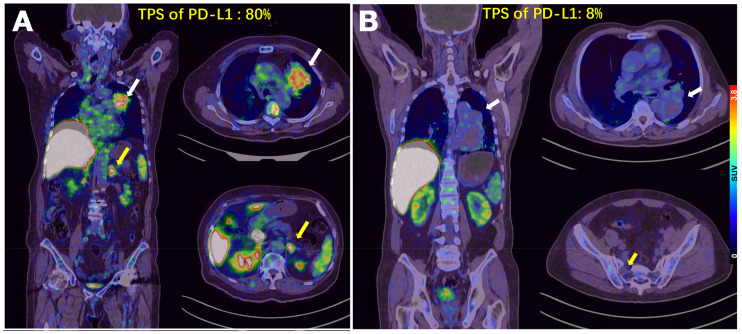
(A) Patient 9, an 80-y-old woman with advanced NSCLC and a PD-L1 TPS of 80%. SUV_max_ of primary tumor was 4.87 (white arrow) and that of left adrenal metastasis was 5.47 (yellow arrow) on ^68^Ga-NOTA-WL12 PET. (B) Patient 3, a 68-y-old man with a PD-L1 TPS of 8%. SUV_max_ of primary tumor in left lung (white arrow) and right sacral metastasis (yellow arrow) were 1.84 and 0.8, respectively, on ^68^Ga-NOTA-WL12 PET.
